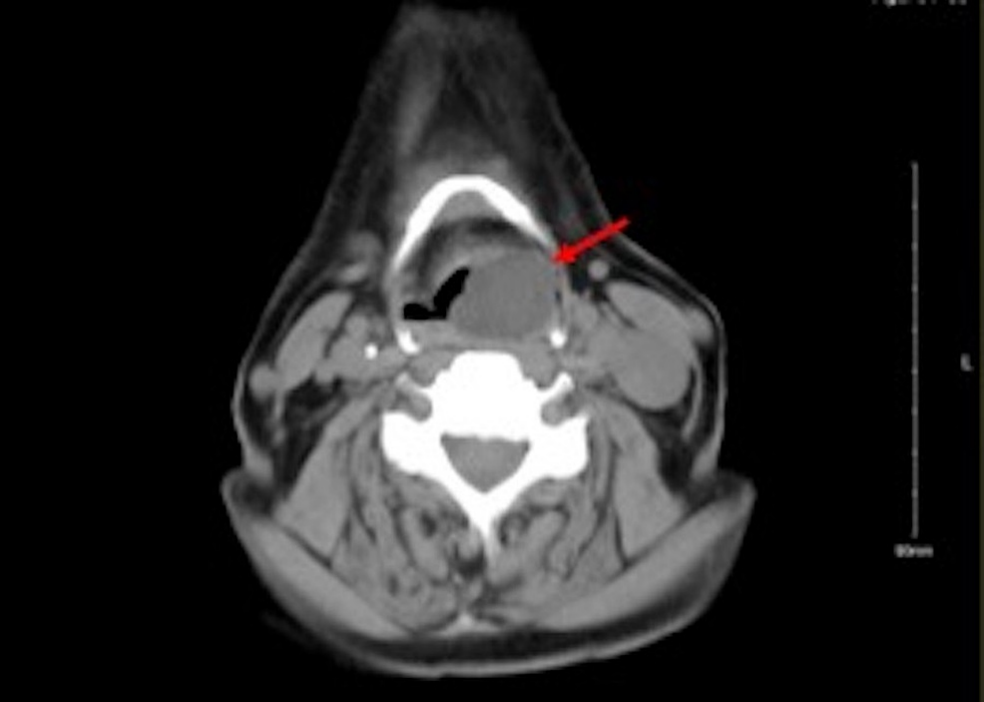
Pre-Op CT scan. Left-sided hypopharyngeal mass with a fatty core.
